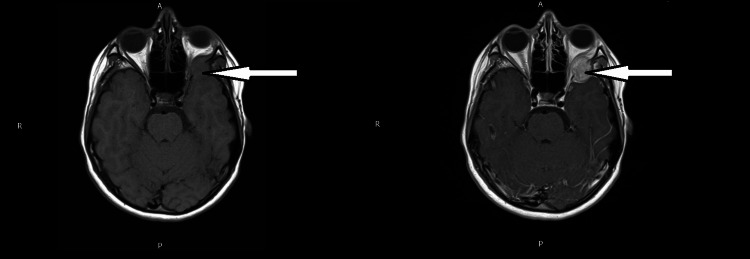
MRI imaging of the head in axial view with T1 pre-gadolinium (left side) and post-gadolinium contrast (right side) Enhancement of hyperintense lytic lesion with gadolinium contrast on the right side as compared to the pre-contrast image on the left side.
